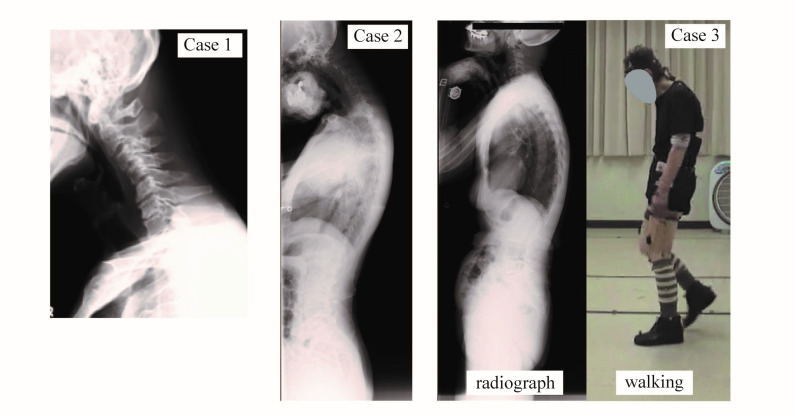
Radiograph images of the cervical spine showing dropped head syndromes of Case 1 (left), Case 2 (middle), and 3 (right). Case 3 managed to correct the cervical alignment momentarily when scanned (left, radiograph) but was not able to maintain the alignment and dropped head was observed during walking (right, photo).
